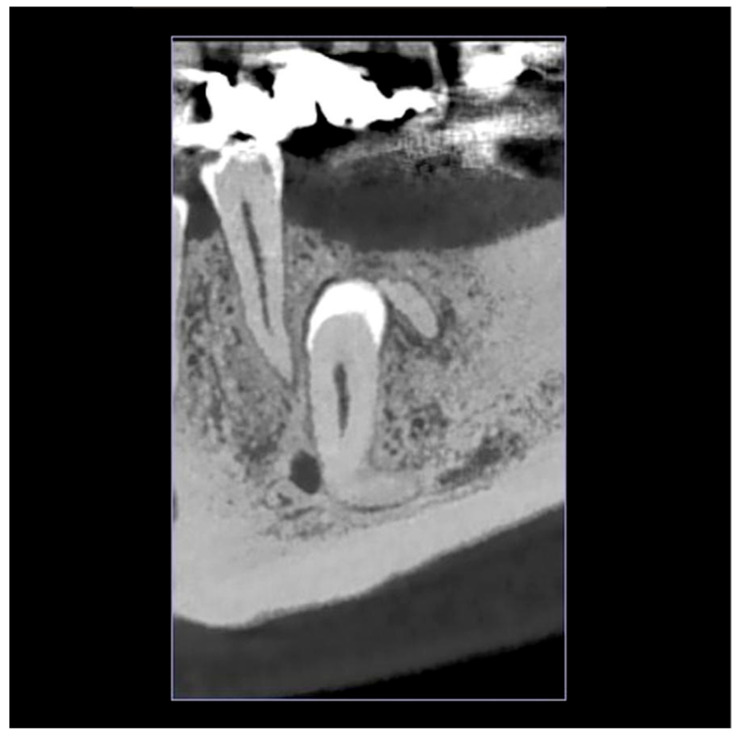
CBCT view showing the impacted second premolar (#35) in proximity to the mental foramen.
